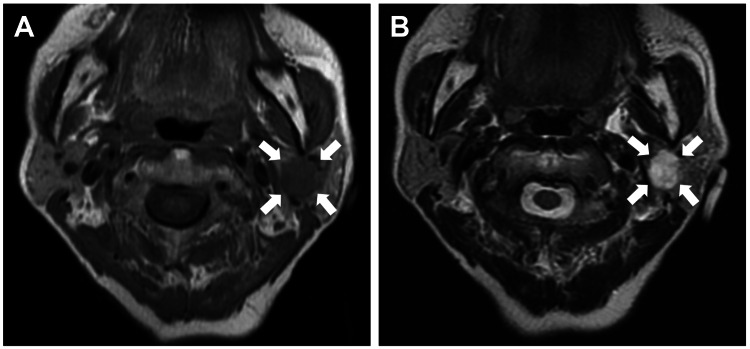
Magnetic resonance imaging (MRI) findings. MRI reveals a well-defined, oval-shaped tumor within left parotid gland on T1-weighted image (A) and T2-weighted image (B).
